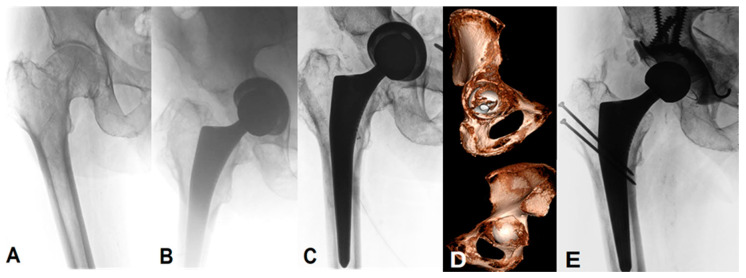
Anteroposterior radiographs and computed tomography images of an 82-year-old man with (A) with a symptomatic primary coxarthrosis on the right side. (B) Intraoperative anteroposterior view during the primary THA with an interruption of the ilioischial and iliopectineal lines. (C) Atraumatic progression of the acetabular fracture with an intrapelvic protrusion of the acetabular component, one day postoperative. (D) CT scan was performed for evaluation of the fracture pattern and the preoperative planning, showing a AAOS type IIB fracture by the presence of an extensive central defect. (E) Postoperative radiograph after the revision with a trochanteric flip osteotomy, a change of the acetabular component and the use of a Ganz reinforcement ring, and a structural and morselized bone allograft.
